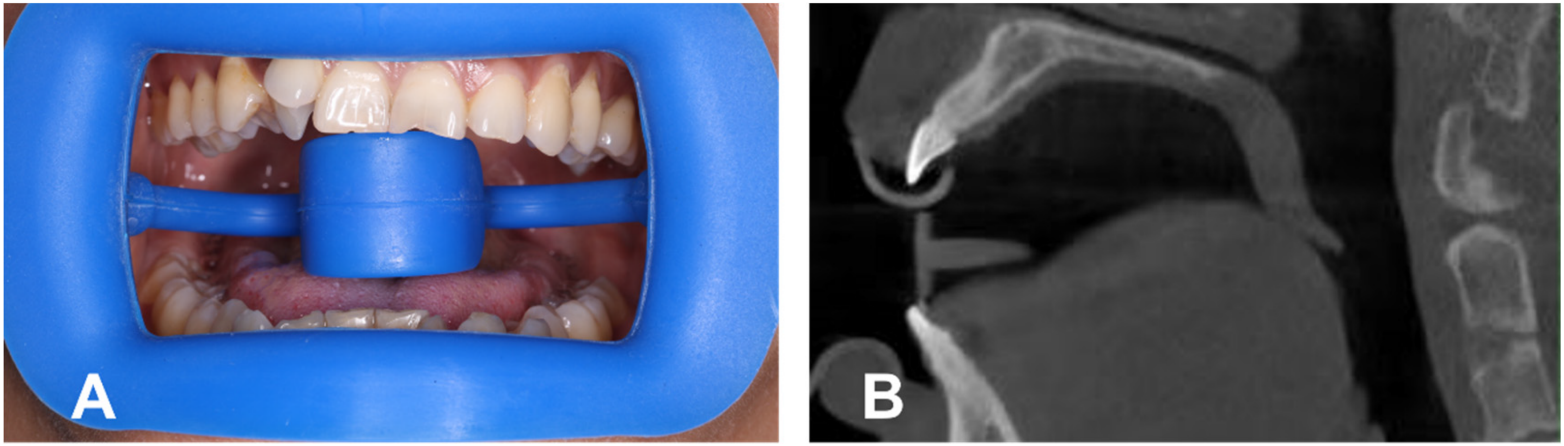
(A) Wearing a mouth opener, with the tongue under the tongue stop. (B) Screenshot of CBCT with the opener.
